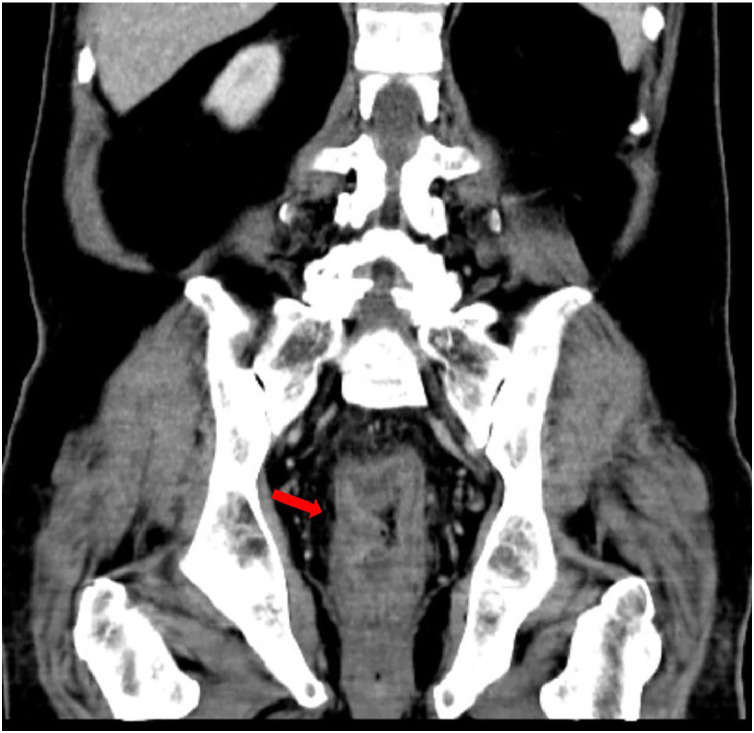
Axial view of abdominal CT scan (A, B), showing long-segment circumferential wall thickening (red arrow), surgical sutures of partial hemicolectomy (blue arrow), and mesenteric engorgement with fat stranding (yellow arrow). Crucially, surgical clips are visible on the superior rectal vein (distal continuation of the inferior mesenteric vein), resulting in its complete occlusion and subsequent venous outflow obstruction (green arrow). Surgical clips located on the inferior mesenteric vein/superior rectal vein complex, resulting in localized venous outflow obstruction.
